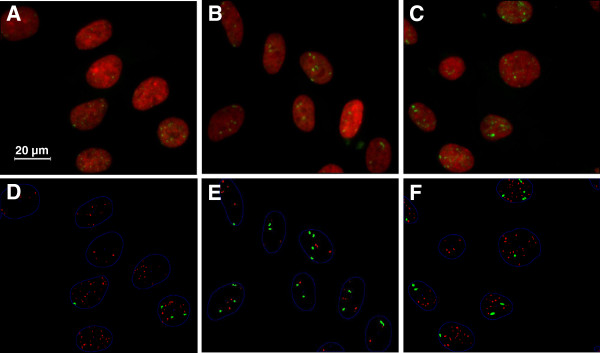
Original and program output sample images for X-ray, mixed beam and alpha-particle irradiated VH10 cells. Images were captured 1 h after irradiation. A-C: Original images. A: 0.20 Gy X-rays, B: 0.27 Gy alpha particles, C: 0.27 Gy mixed beams (0.20 Gy X-rays + 0.07 Gy alpha particles), D-F, corresponding program output images. D: Analysis image from A, X-rays. E: Analysis image from B, alpha particles. F: Analysis image from C, mixed beams. In images D-F: red dots = small foci, green dots = large foci.
